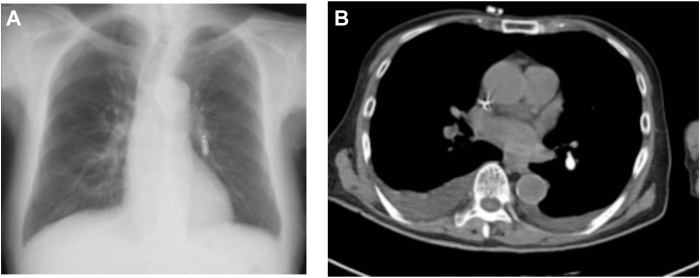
A: Chest radiograph showing the dislodged Micra pacemaker (Medtronic, Tokyo, Japan) in the left pulmonary artery (LPA). B: Computed tomography image showing the Micra pacemaker embolized into a branch of the LPA.
